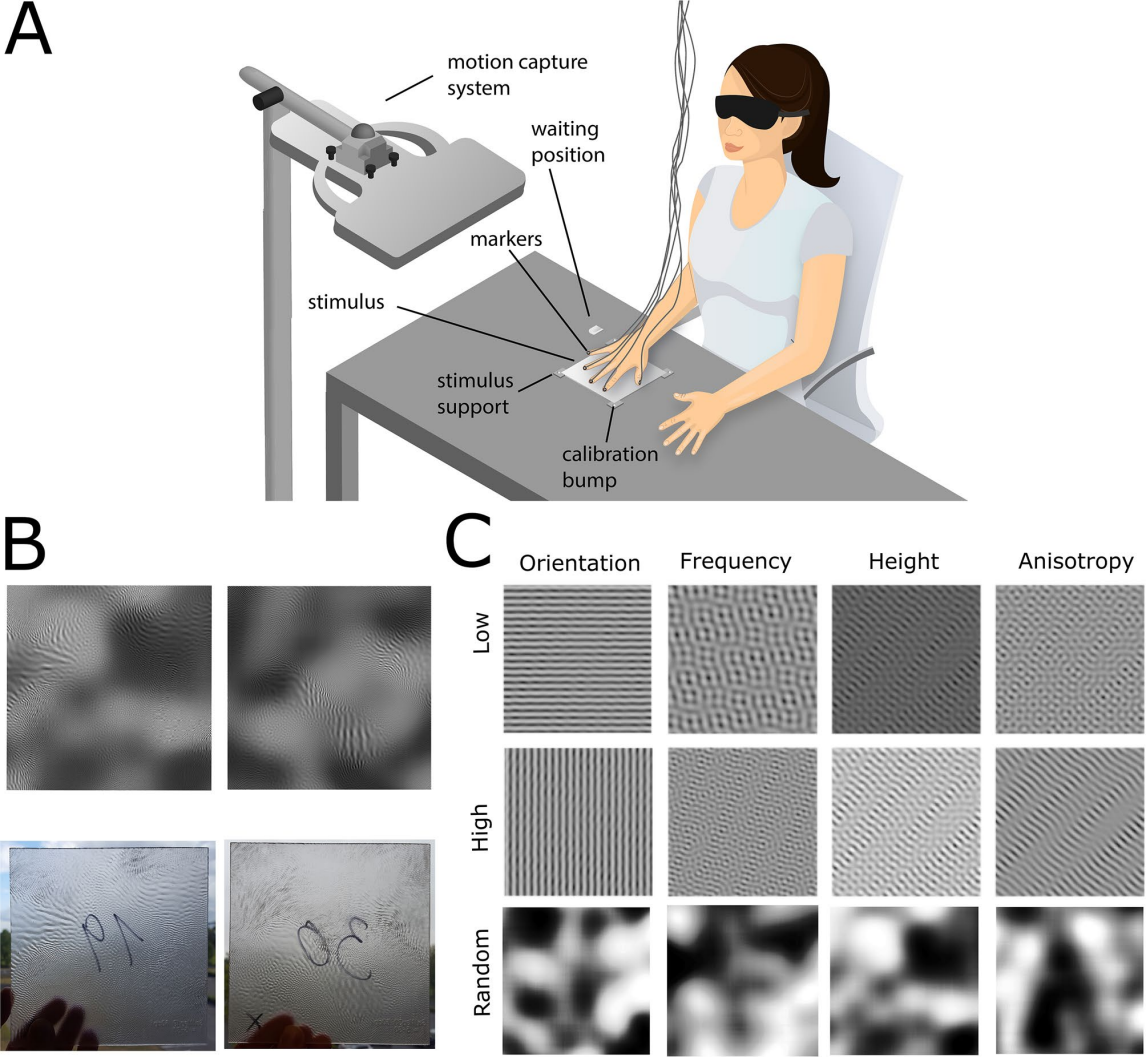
(A) Setup. Participants sat at a table together with the experimenter who positioned the stimuli in front of them. Stimuli were stabilized at the four corners. The position of each finger of the participant’s right hand was tracked with the ultrasound based motion tracking system (Zebris). Prior to the experiment the position of the right index finger was calibrated using the calibration bumps in the stimulus support corners. Between trials participants moved the hand to the waiting position marked as a finger-holder for the middle finger. (B) Example stimuli. Surface reliefs on top with their printed versions below. (C) Stimulus features used to create the stimuli: orientation direction (Orientation), spatial frequency (Frequency), surface relief height (Height) and spatial anisotropy (Anisotropy). The features are exemplified by uniform stimuli (not used in the experiment), in which all features are kept constant but one is set either to the minimum (“Low”, first row) or the maximum (“High”, second row) of the corresponding feature range. The third row (“Random”) shows four pink noise maps, one per feature, used to generate the example stimulus 19 in B).
